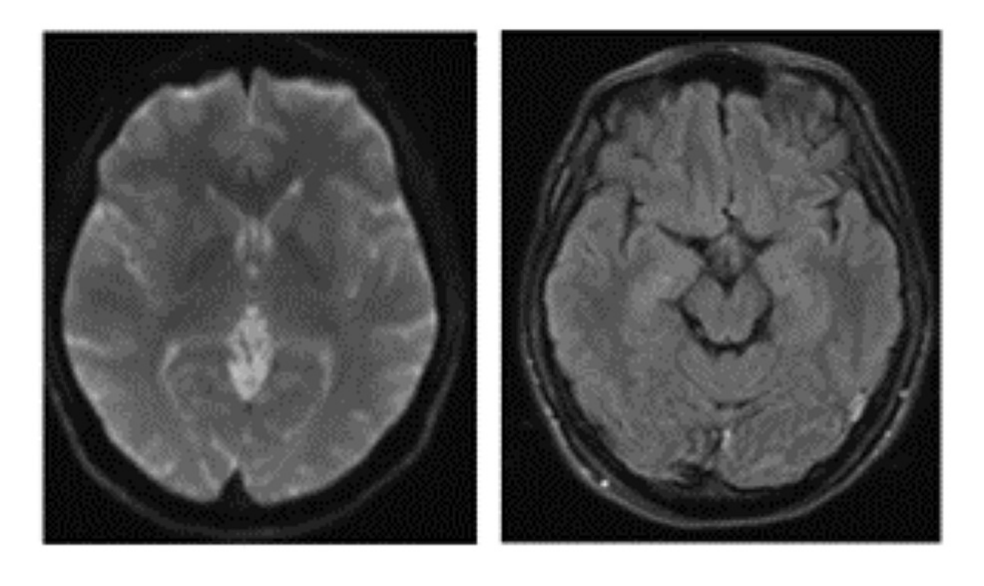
Magnetic resonance imaging of the brain showing T2 FLAIR and diffusion-weighted imaging sequences with no acute intracranial hemorrhage, acute infarct, or intracranial space-occupying lesion. There is no pathological intracranial contrast enhancement. FLAIR: fluid-attenuated inversion recovery
